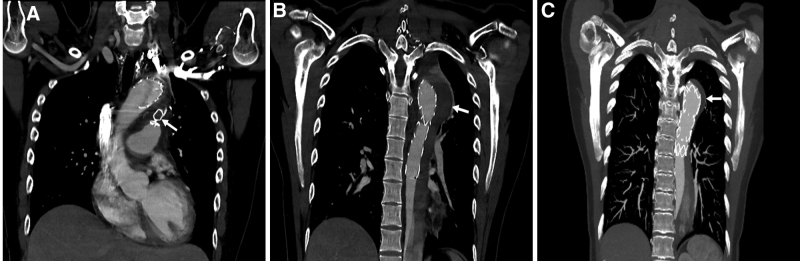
(A and B) Coronal CT performed 3 days after PDA embolization showing a normally positioned vascular plug (arrow in A) in PDA and a false lumen filled with a thrombus (arrow in B) within the PDA. (C) Coronal CT performed 6 months after PDA embolization showing a successfully remodeled and shrunken false lumen (arrow). CT = computed tomography, PDA = patent ductus arteriosus.
